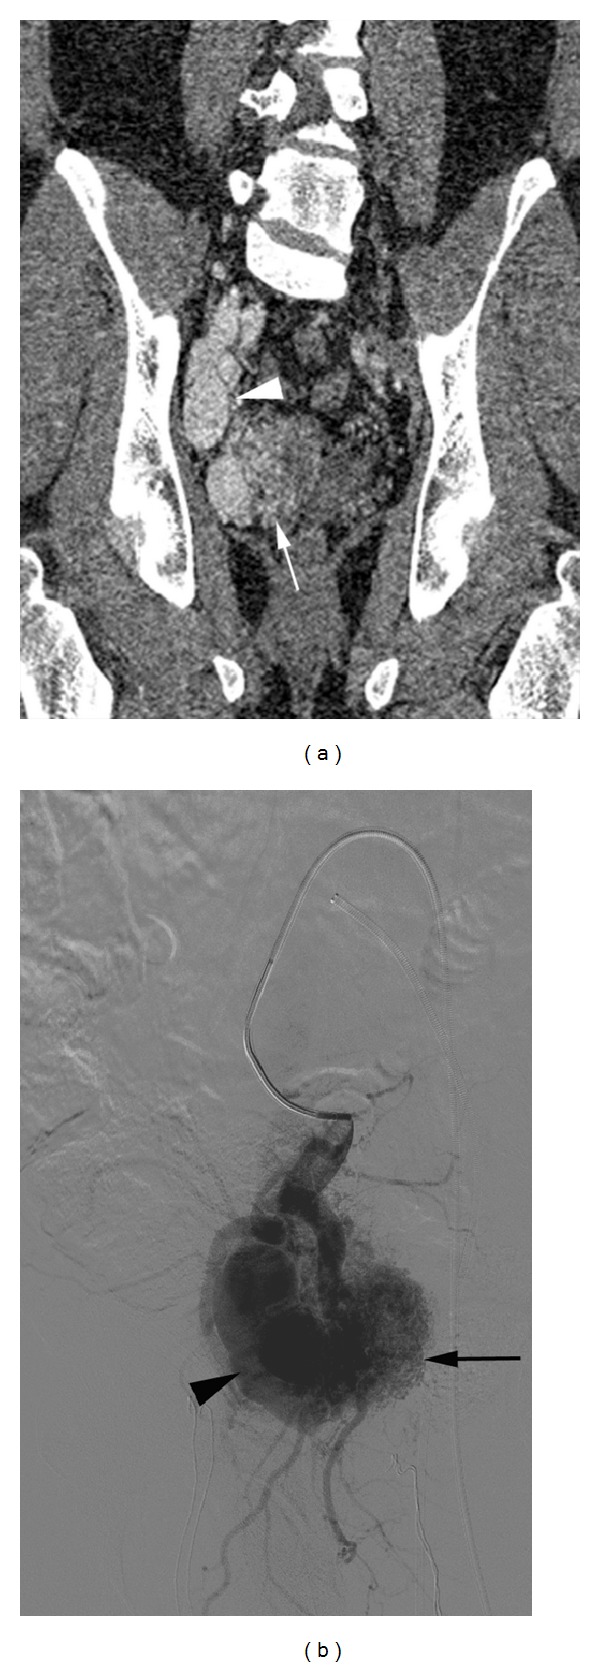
(a) Contrast-enhanced computed tomography in the arterial phase revealed a large pelvic arteriovenous malformation in the right lower quadrant. Multiple fine striations representing multiple feeding arteries (arrow) with early enhancement of a large venous pouch (arrowhead). (b) Selective arteriography of the right internal iliac artery anterior trunk revealed the nidus (arrow) with multiple fine feeders, dilation of the draining vein (arrowhead), and early drainage of the right internal iliac vein.
